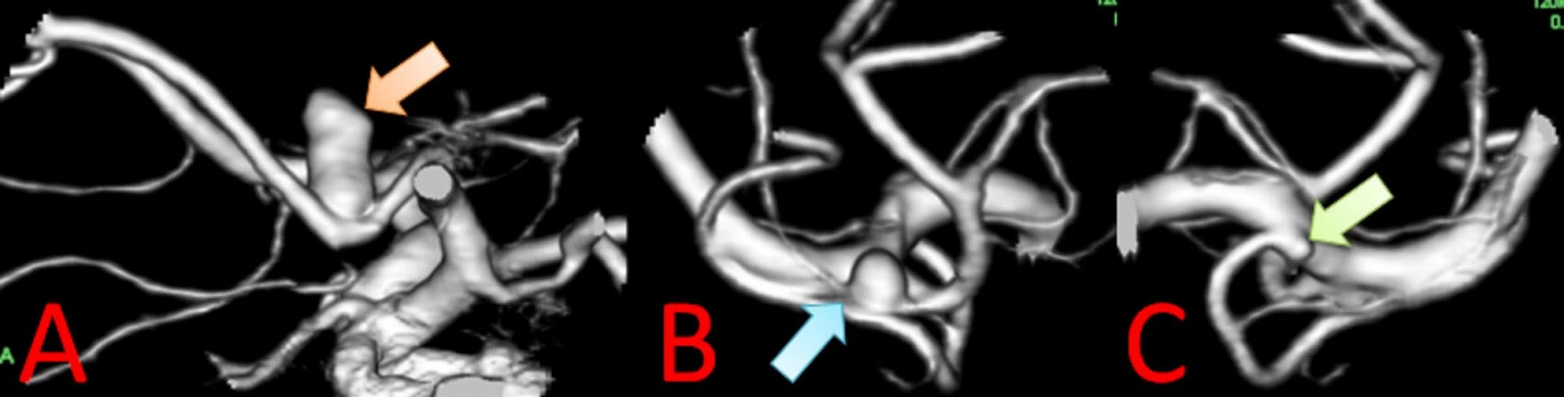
Preoperative contrast-enhanced 3D CT findings of cerebral aneurysms. A An anterior communicating artery (Acom) aneurysm (10 mm, orange arrow) with a bleb. B A right middle cerebral artery aneurysm (3 mm, blue arrow). C A right M1 bifurcation aneurysm (1.5 mm, green arrow)
